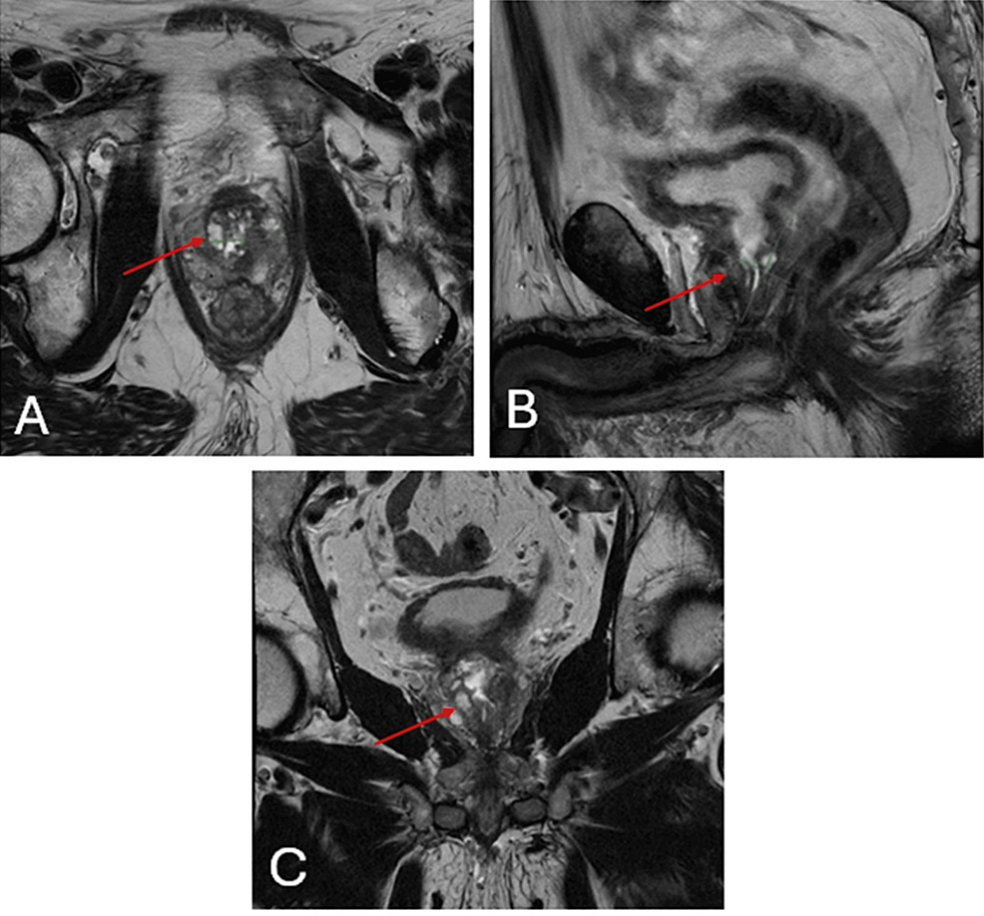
Axial (A), sagittal (B), and coronal (C) views of the MRI pelvis demonstrate enlargement of the prostate (red arrows), measuring 4.2 x 3.2 x 3.9 cm. MRI: magnetic resonance imaging
